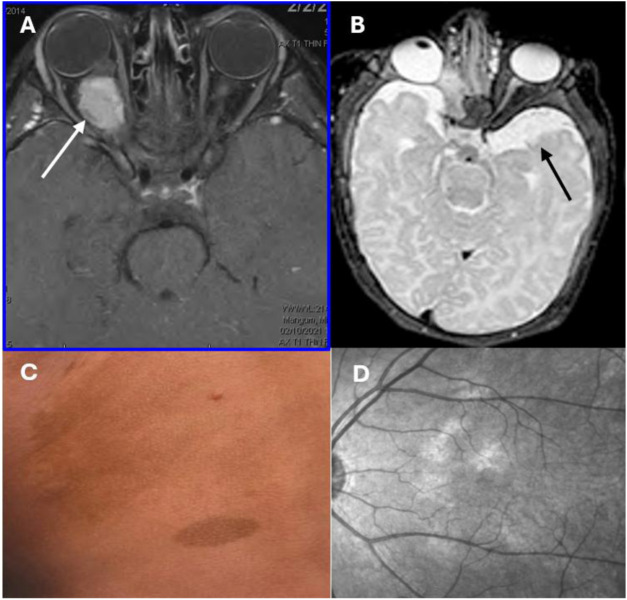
Representative ocular and systemic findings in neurofibromatosis type 1. (A) Optic pathway glioma on MRI. (B) Sphenoid bone dysplasia. (C) Cutaneous neurofibroma and Café-Au Lait spots. (D) Choroidal nodules visualized on Near InfraRed OCT.
